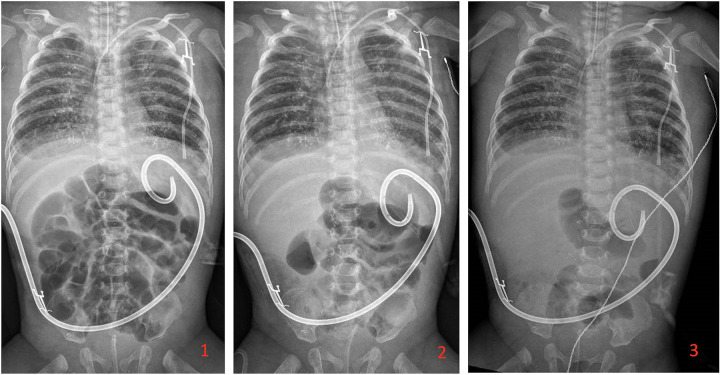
Serial radiographic imaging following attempted thoracic duct sclerotization. Fluoroscopic images obtained 1–2 and 4 days after the interventional radiology procedure demonstrate persistent failure to opacify the thoracic duct, confirming the unsuccessful outcome of the sclerotization attempt.
